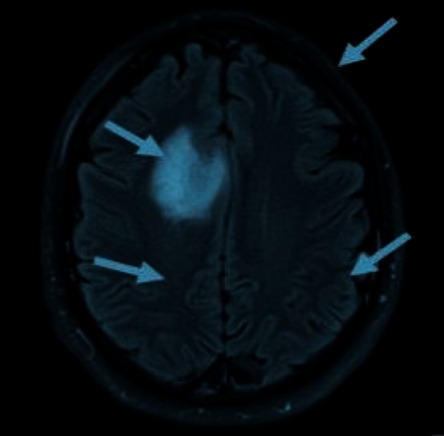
MRI image of brain tumor.
